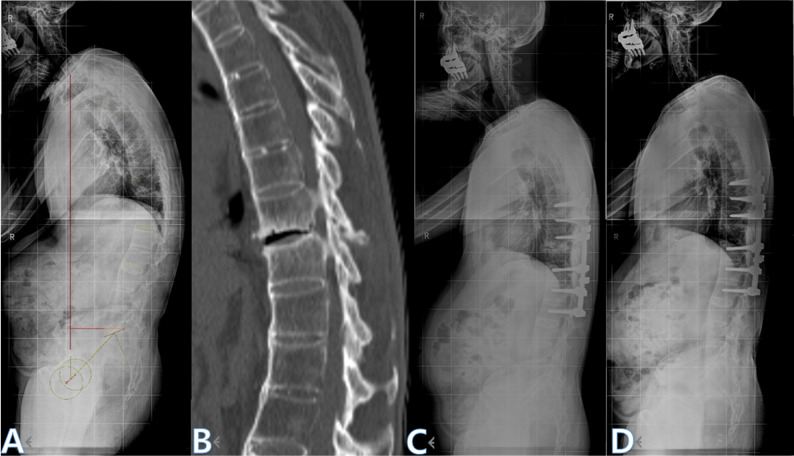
Representative case of an intervertebral space (IS-type) fracture treated with PSF. A Preoperative lateral radiograph showing kyphotic deformity. B CT scan demonstrating a disc space fracture at T11–12. C Radiograph at 3 months postoperative showing realignment after PSF. D Final follow-up (2 years) radiograph showing union and maintained correction
